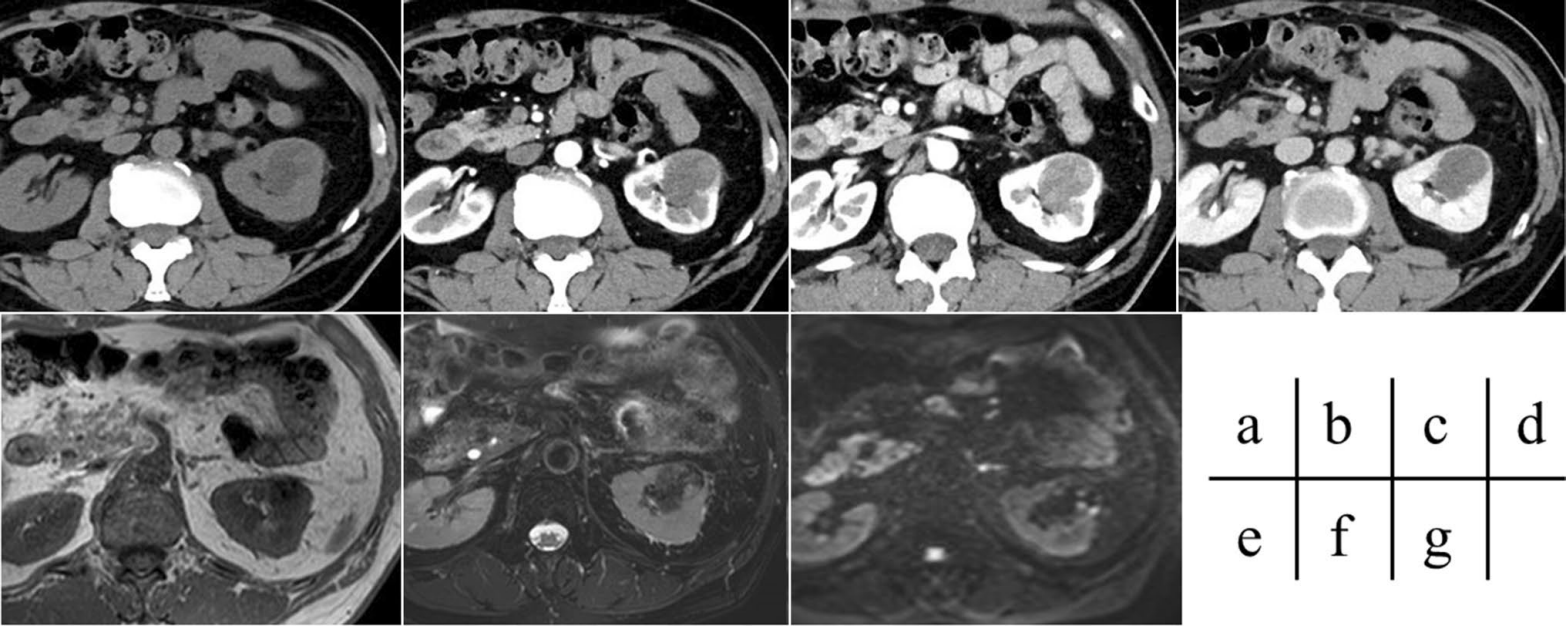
Images from a 72-year-old man with unclassified RCC. A mass lesion with expansive growth is identified in the left kidney. The tumor appears hypodense on unenhanced CT (a) and exhibits a mild washout pattern on DCE imaging (b–d). The tumor appears mildly hypointense on T1-weighted imaging (e) and hypointense on T2-weighted imaging (f) with suspected hemorrhage and hypointense on DWI (g). A partial pseudocapsule is identified. Unclassified RCC was diagnosed after surgery. As of postoperative Day 2462, the patient remains free from recurrence
